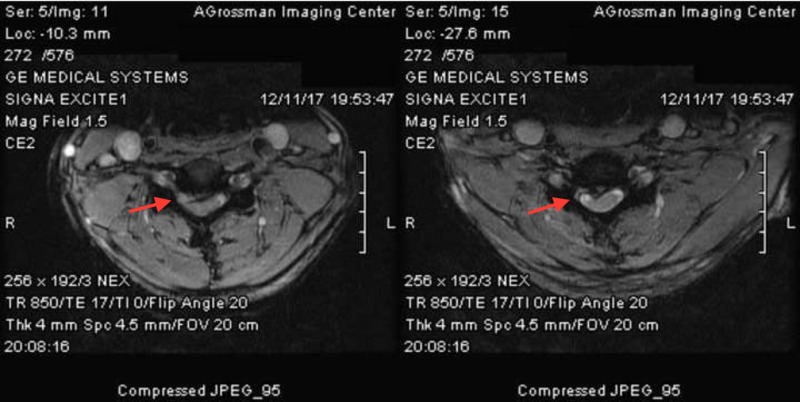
Axial MRI of cervical spine Retrolisthesis with 5 mm right paracentral disc herniation extending into the foramen (left) and C5-6. A right paracentral disc extrusion compressing the right anterior aspect of the spinal cord (right).
